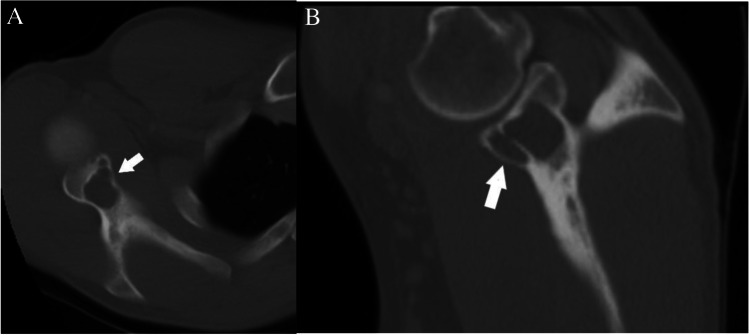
Case 9. Subchondral cyst. 30-year-old man presents with 1 month of pleuritic chest pain. There is an asymptomatic incidental lucent lesion (arrows) in the glenoid which abuts the articular surface on the A axial and B sagittal CT images. Lesion has mean density of 25 HU
